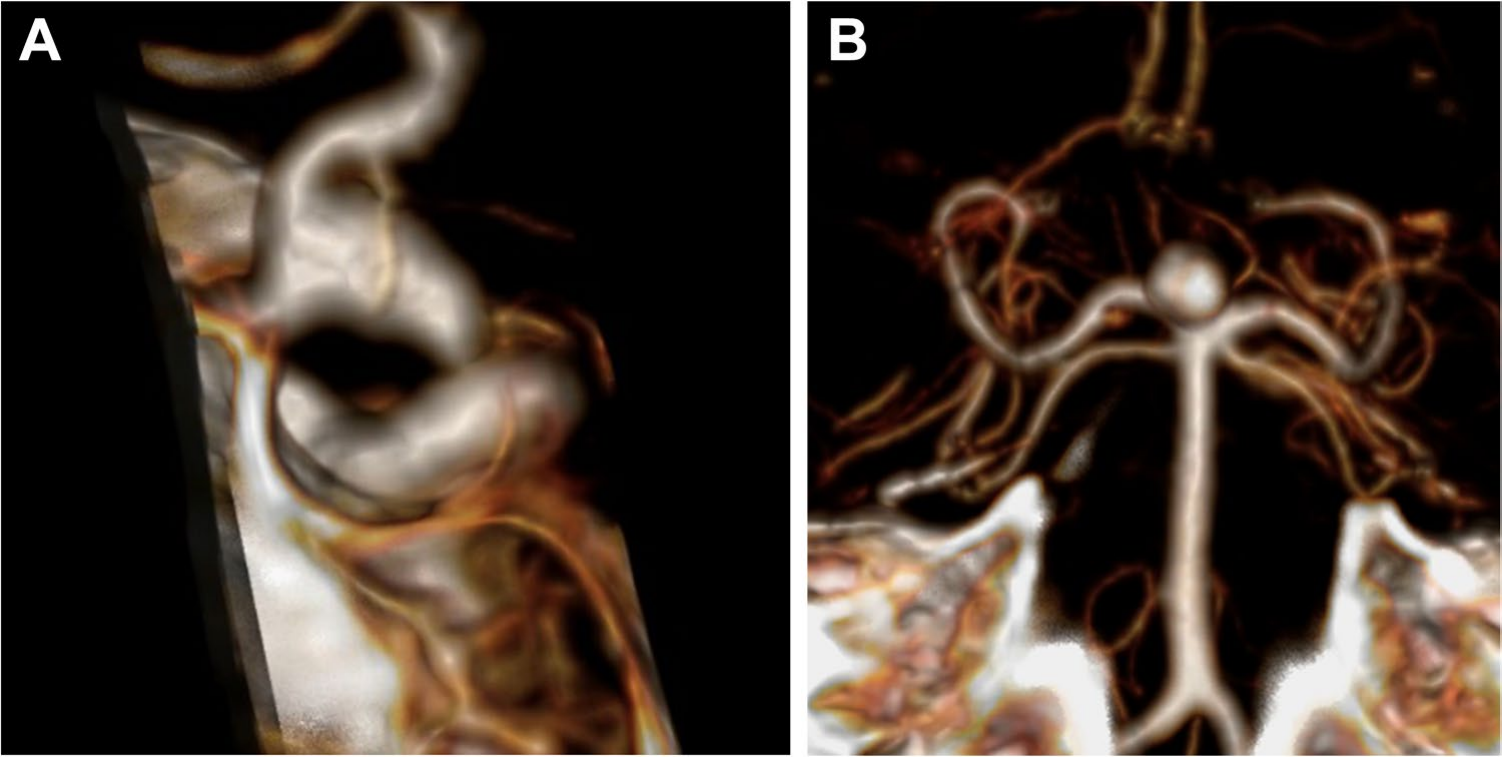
CT 3D reconstruction showing the basilar tip unruptured aneurysm in (A). sagittal and (B). coronal views
